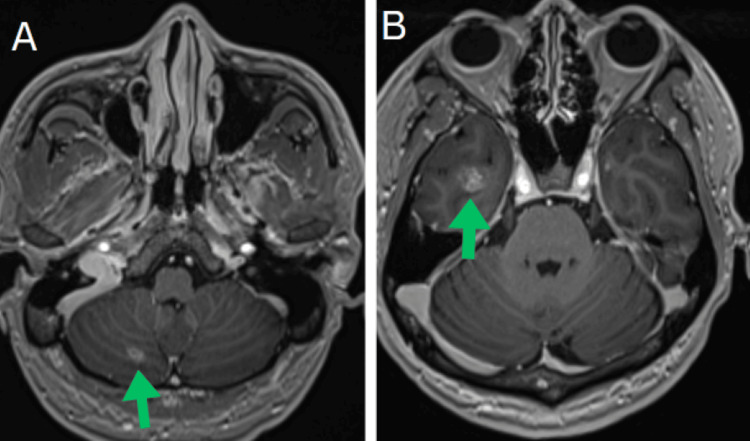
Initial brain magnetic resonance imaging (MRI) images. A: 7 mm enhancing lesion is present in the right inferomedial cerebellum. B: 1.1 cm enhancing lesion is seen superficially in the right inferior temporal lobe, accompanied by mild surrounding vasogenic edema and subtle petechial hemorrhage.
